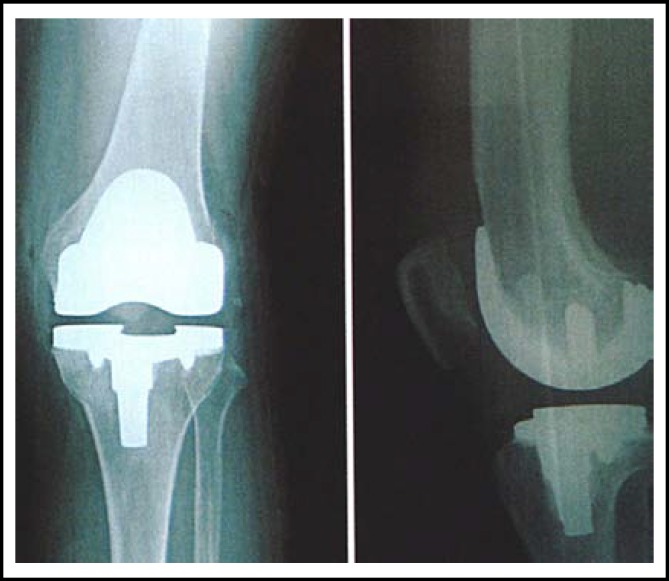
Lateral knee X-ray after surgery disclosed that there were no radiolucent regions at the interface between prosthesis and bone cement or that between bone cement and bone, and the prosthesis was firmly fixed in the ideal position.
